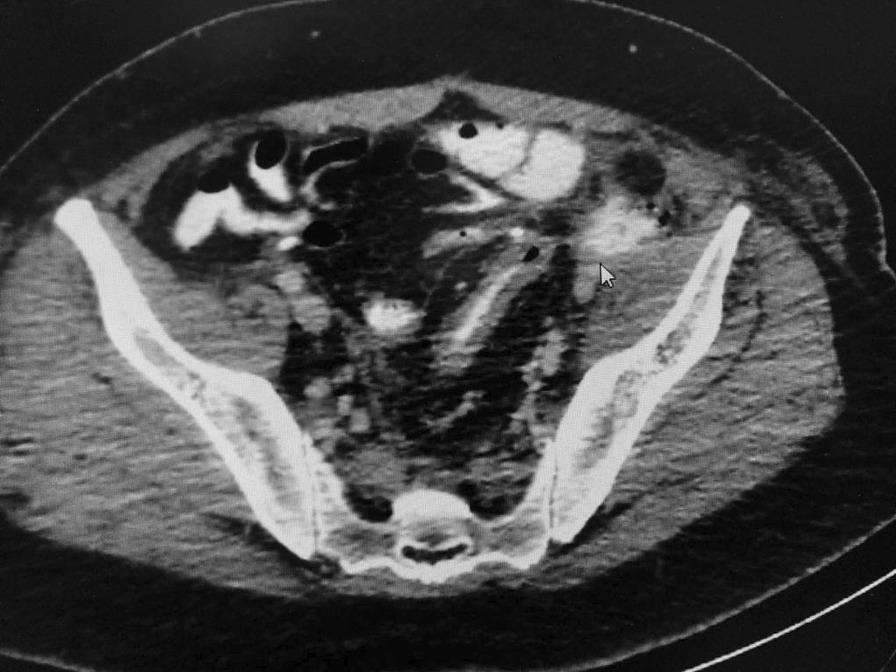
Sagittal CT showing fluid collection seen in the left iliac fossa anterior to the left external iliac artery
